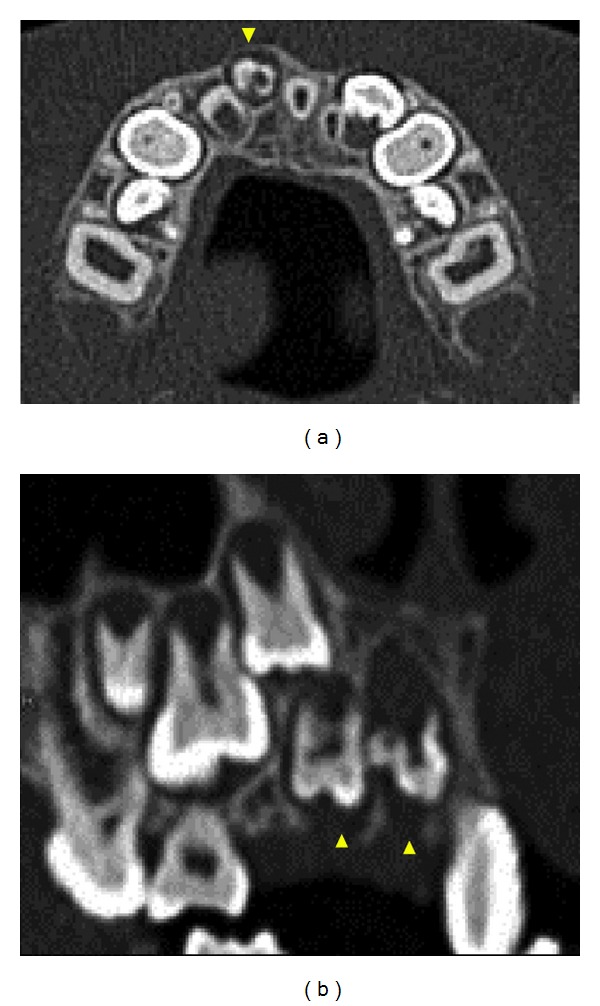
CT images of case 2. (a) Axial CT image shows labial expansion of dental follicles (arrowheads). (b) Multiplanar reconstruction image shows dental follicles clearly expanding toward alveolar ridge (arrowheads).
